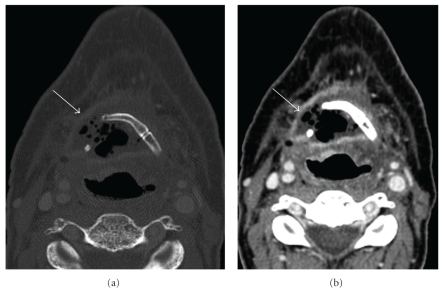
Hyoid bone osteoradionecrosis: (a) Axial contrast enhanced CT of the mandible bone (bone window) shows destruction of the right hyoid bone with soft tissue air (arrow). (b) Axial contrast-enhanced CT (soft tissue window) shows soft tissue ulceration without evidence of an enhancing soft tissue mass (arrow).
